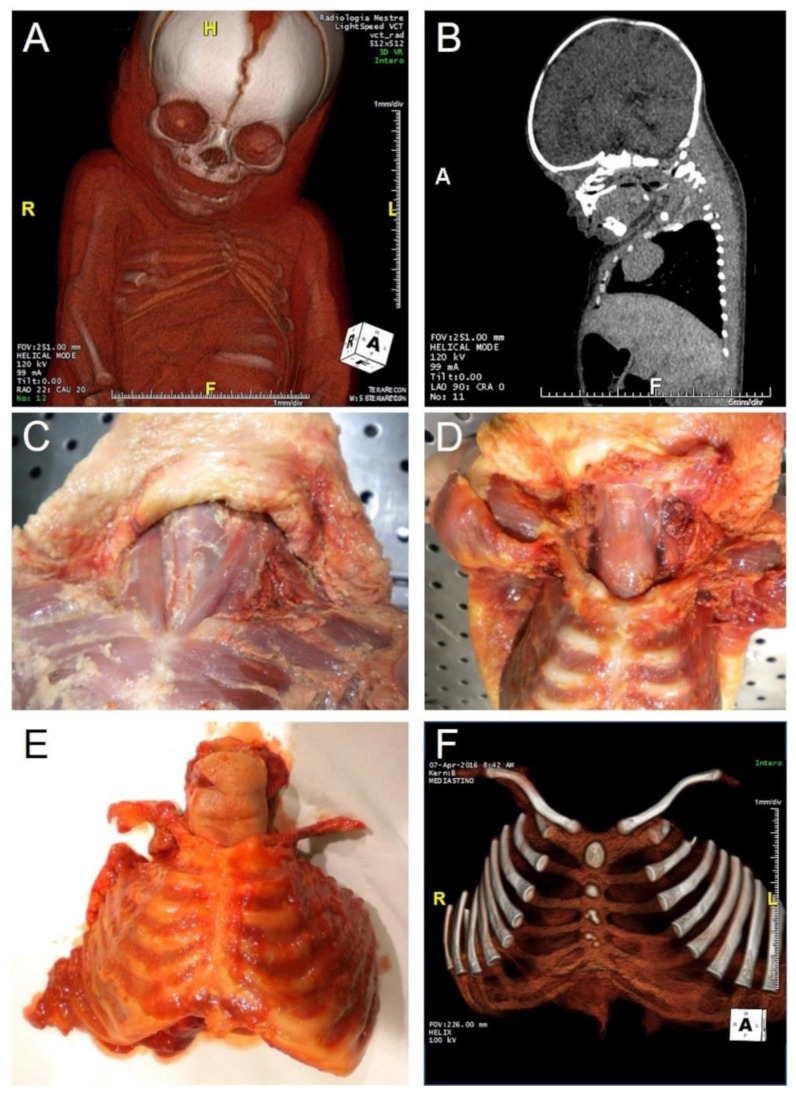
Infant reported to be found dead in the parents’ bed: accidental or abusive trauma? Sudden Infant Death Syndrome? Undiagnosed pathology? (A,B) post-mortem computed tomography, with radiologist’s description of thorax deformation. (C,D) anatomical dissection of the neck and thorax, showing the absence of hemorrhagic infiltrations or fractures/dislocations. (E) Sampling of the whole anterior thoracic wall. (F) post-autopsy computed tomography of the anterior thoracic wall, confirming the absence of traumatic findings. The radiological misdiagnosis was ascribed to artifacts due to previous corpse freezing in an anomalous position. (H: Head; F: Feet; L: Left; R: Right).
